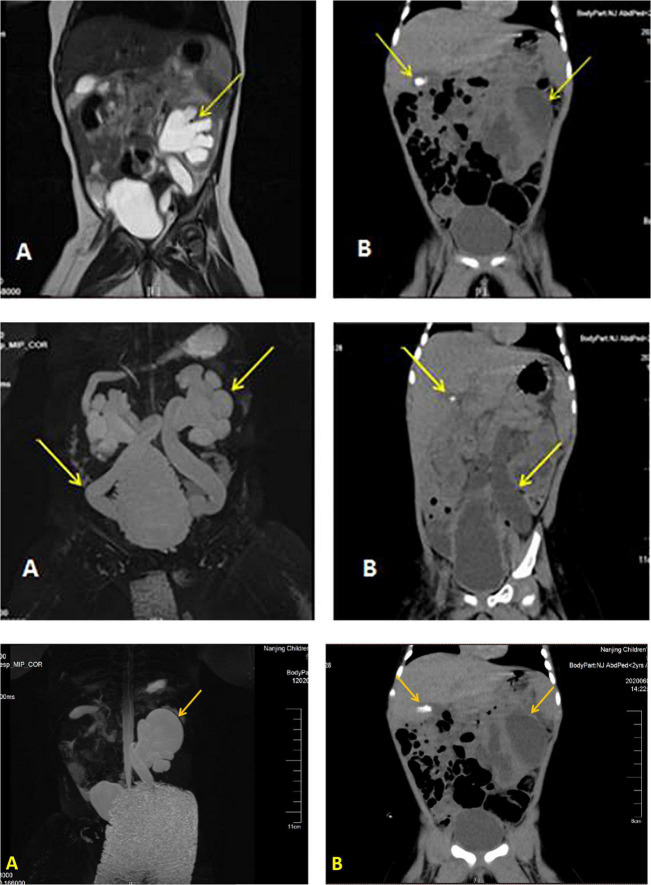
Imaging results displaying a dilated left ureter and renal pelvis and gallstone in the gallbladder. Image of the urinary MR of case 1 (A), Full abdominal CT of case 1 (B).
